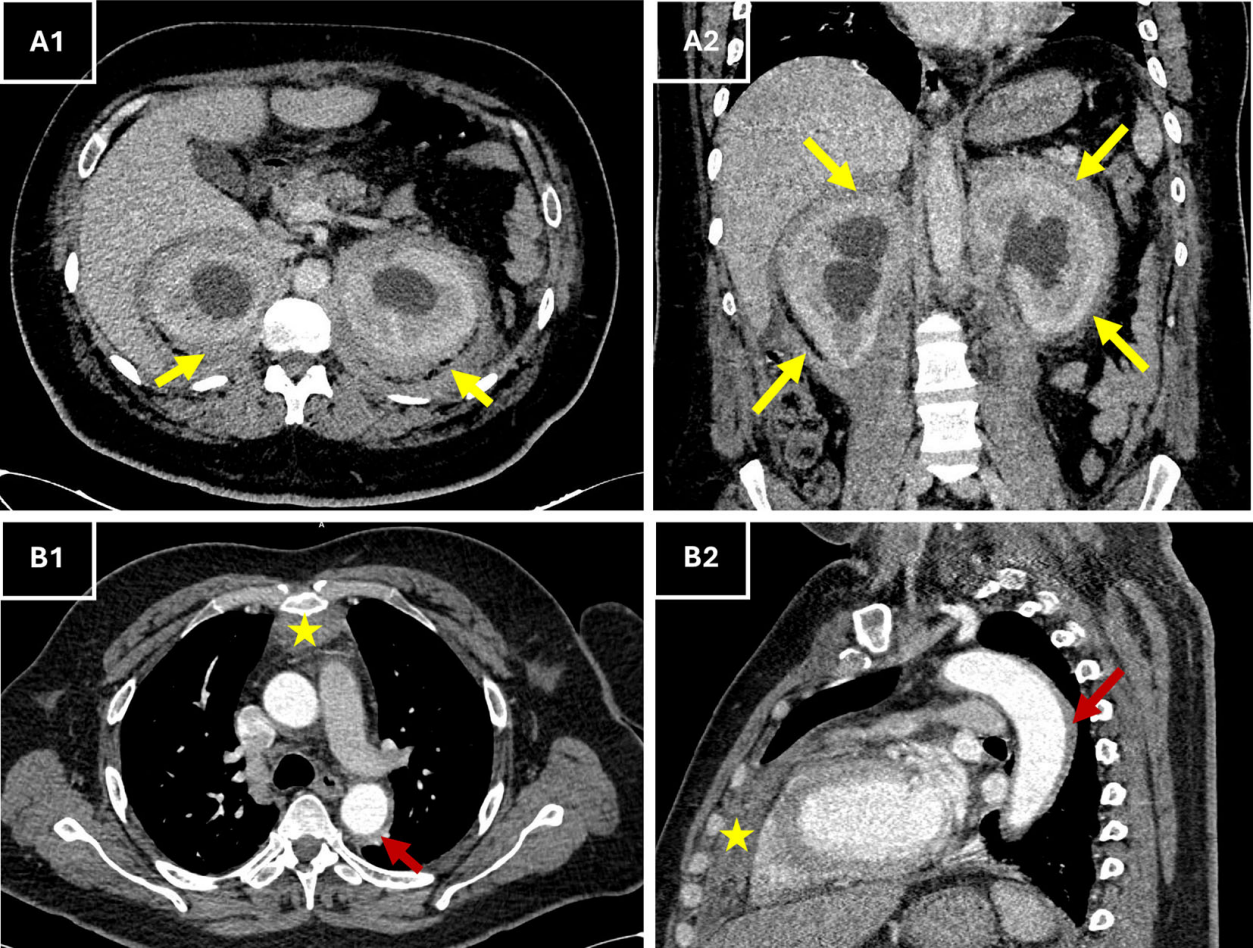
Contrast-enhanced CT images showing characteristic abdominal and thoracic involvement in ECD. (A1–A2) Axial (A1) and coronal (A2) contrast-enhanced CT views showing circumferential, bilateral perirenal soft tissue thickening (yellow arrows) consistent with the “hairy kidney” sign. (B1–B2) Axial (B1) and sagittal (B2) CT views showing retrosternal anterior mediastinal soft tissue thickening (*, red arrows) extending to the pericardium, in keeping with pericardial and mediastinal involvement described on the report. Abbreviations:
